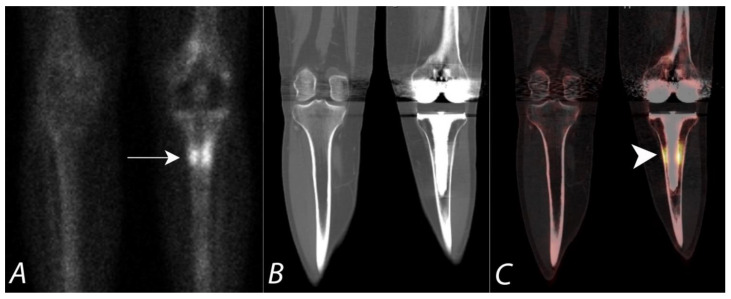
Fifty-year-old female with a painful left knee prothesis. Anterior planar (A), coronal CT (B) and coronal SPECT/CT (C) images of a Tc-99m MDP bone scan of both knees demonstrate a prominent increased uptake in a band surrounding the midportion of the left tibial prosthetic stem. Plain AP radiographs demonstrate sclerosis without periprosthetic lucency. Findings are consistent with being related to altered biomechanical remodeling (similar to a stress fracture). There is no evidence for loosening or infection.
